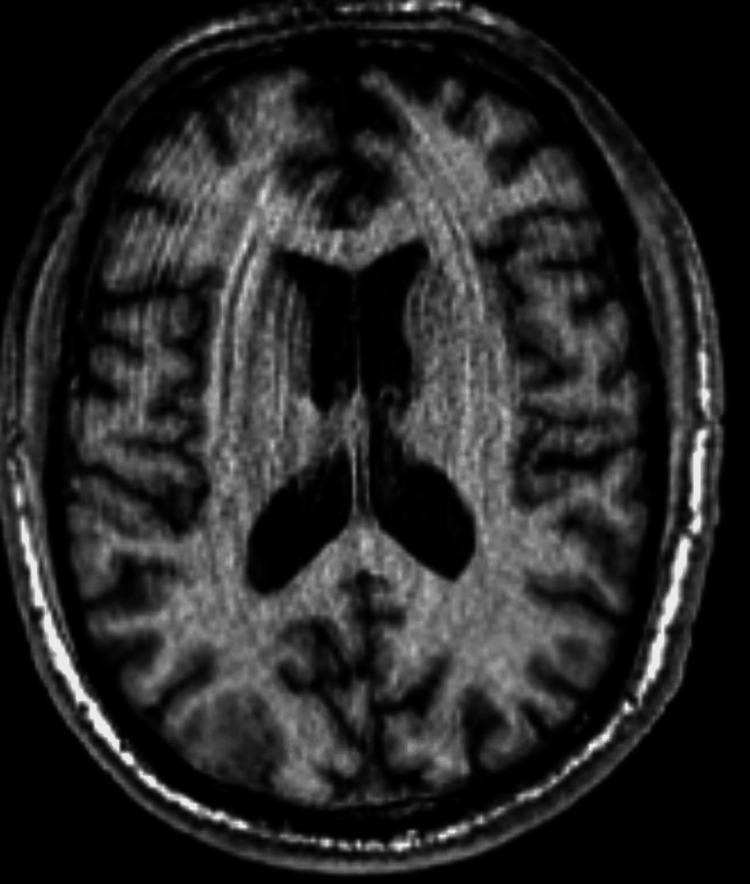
MRI with contrast (no significant findings)
